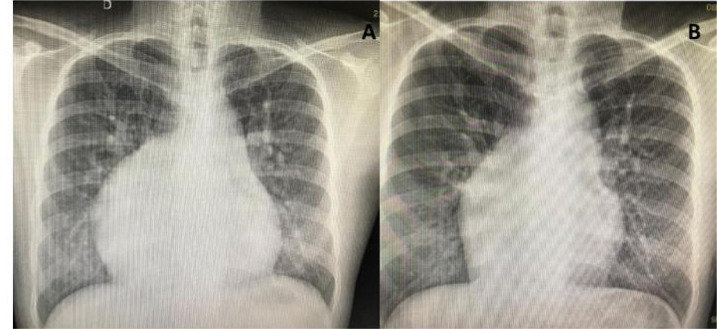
Preoperative (A) and three-year postoperative (B) chest radiographs
